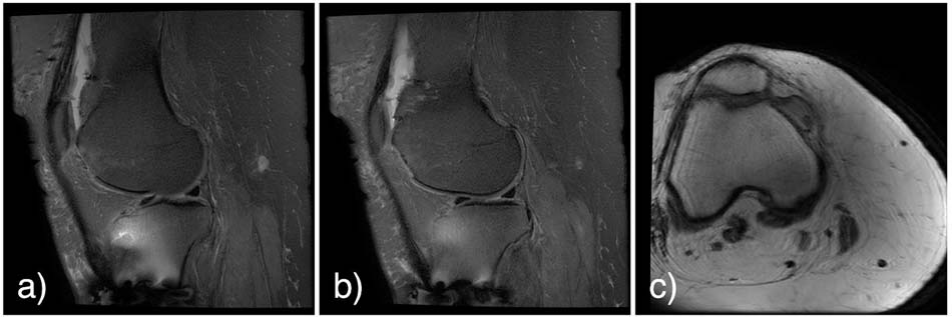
Noncontrast multiplanar T1 (a and b) and T2 (c) MRI at 7 weeks post-op showing a large full-thickness defect on the lateral femoral trochlea measuring 1.8 cm in diameter with a loose chondral fragment in the superior medial joint space.
